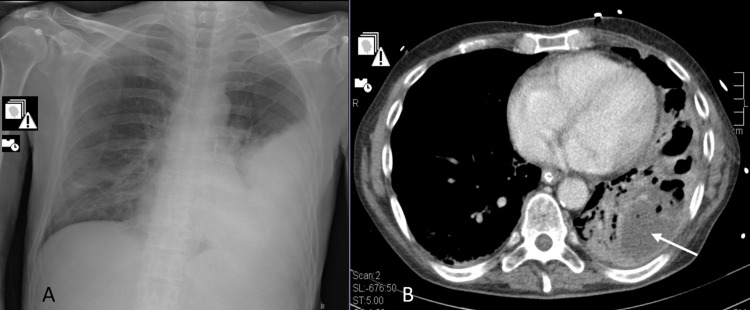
X-ray and CT scan of the patient in case 2 A: X-ray at admission - left lower consolidation. B: CT scan made on day 10 of hospital stay showing a lung abscess and cavitation.
